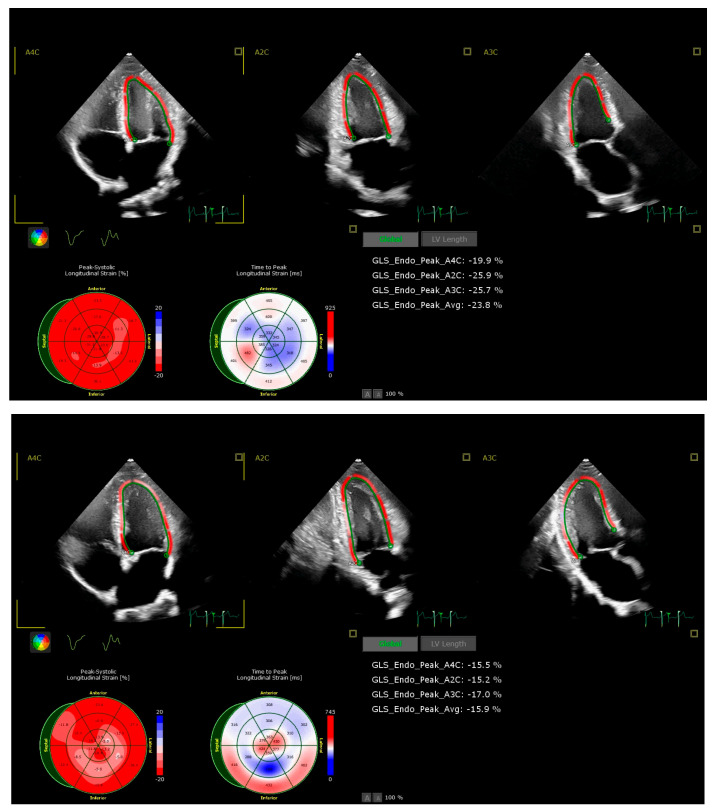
Examples of echocardiographic assessment of LV GLS in an untrained participant at rest (upper panel, GLS −23.8%) and post-exercise (lower panel, GLS −15.9%) in hypoxic conditions.
